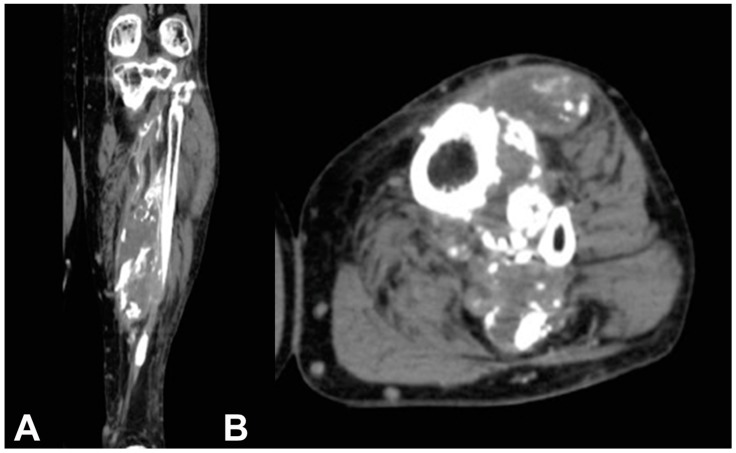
(A) Coronal and (B) axial CT scan of the leg show a calcified fusiform soft-tissue mass with peripheral plaque-like calcification linearly oriented through the muscles of the anterior and posterior leg compartment and interosseous membrane and marked erosion of the fibular cortex. The scalloping of the tibia suggests a slow growing process; central low signal density, and peripheral high density suggests fluid and peripheral calcification.
